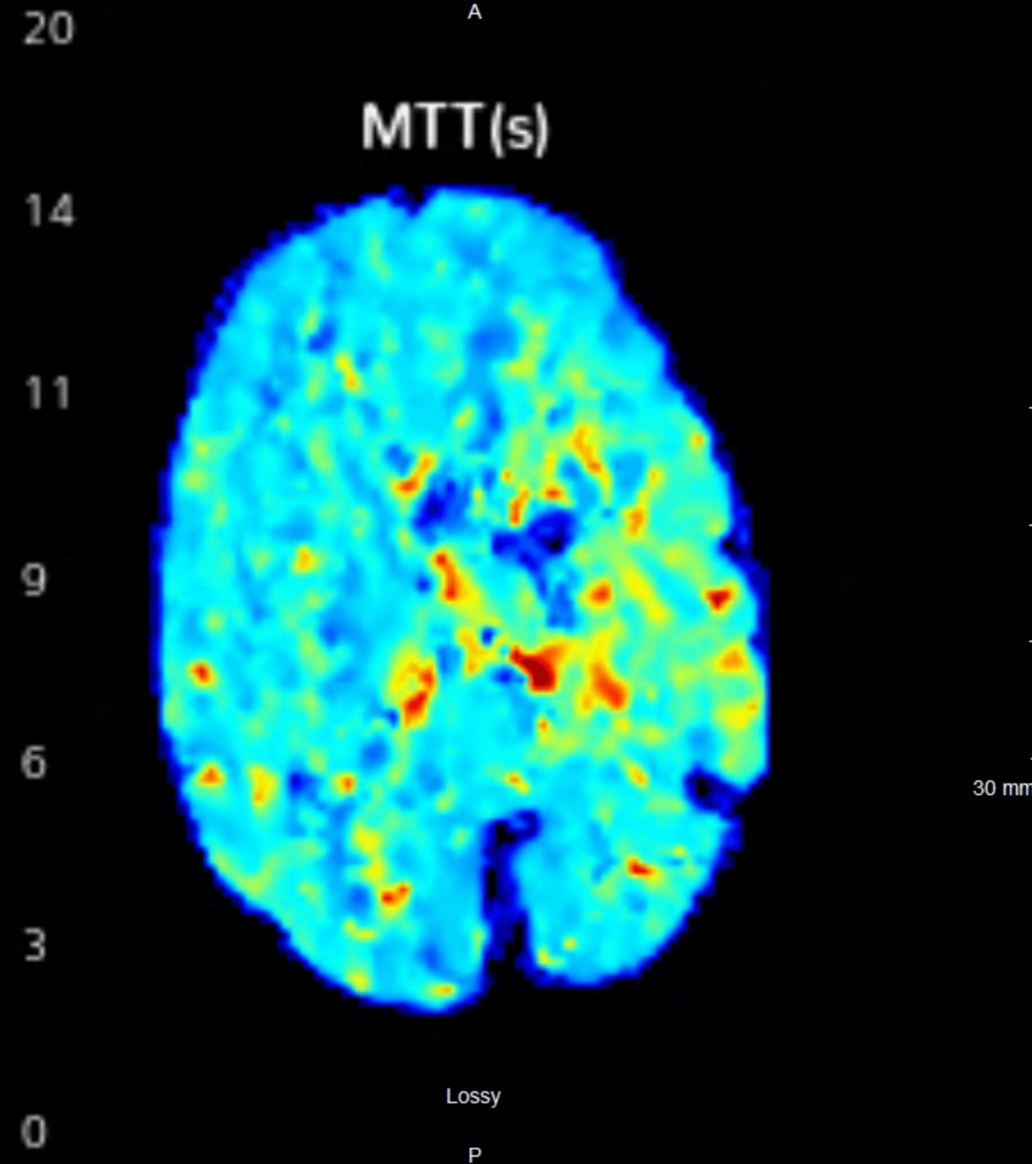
CT perfusion showing mismatch perfusion defect in left frontal parietal region. MTT: mean transit time
